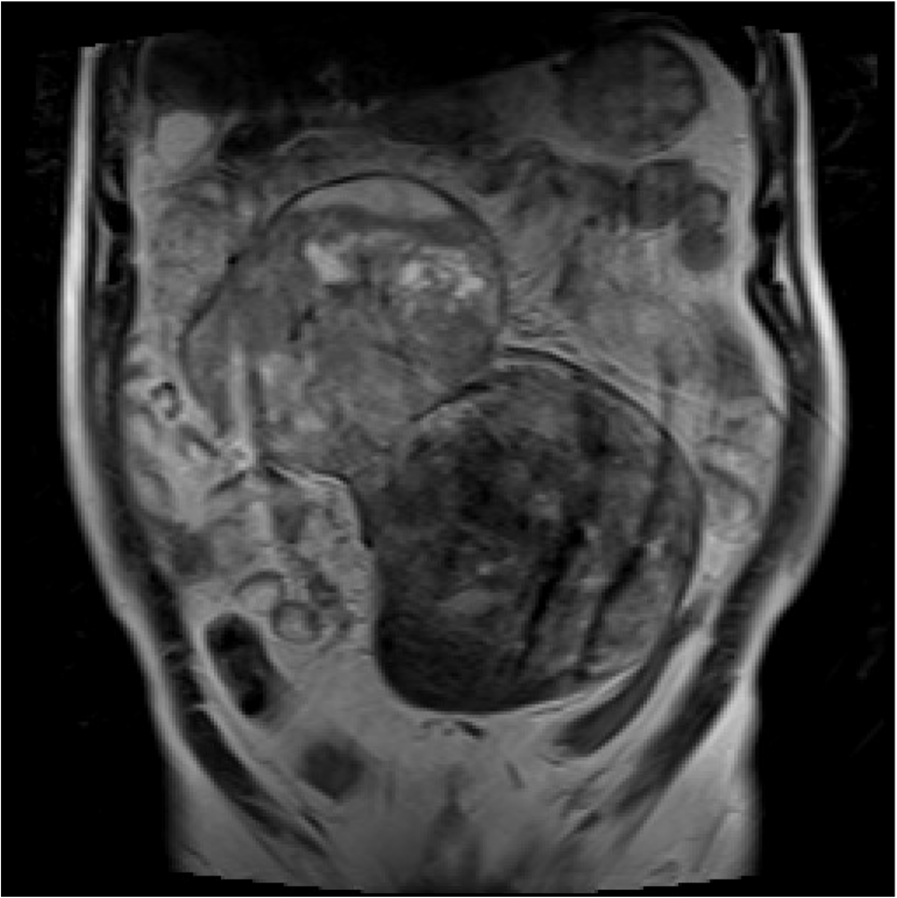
Magnetic resonance imaging demonstrated a well-circumscribed mass with a heterogeneous high signal intensity on T2-weighted imaging.
